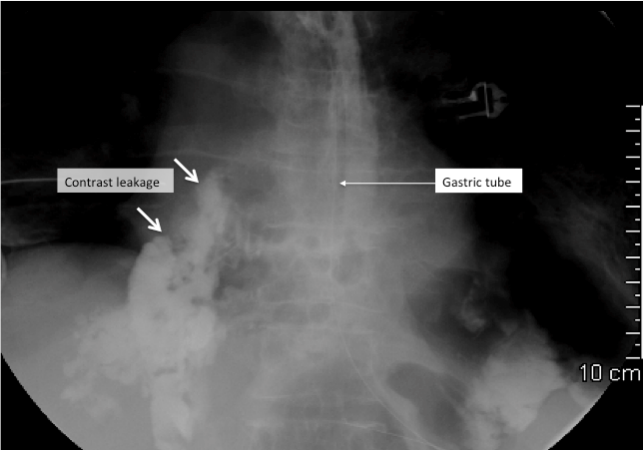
Plain chest radiography with a water soluble contrast swallow, showing contrast leakage in a patient with spontaneous rupture of the esophagus.
